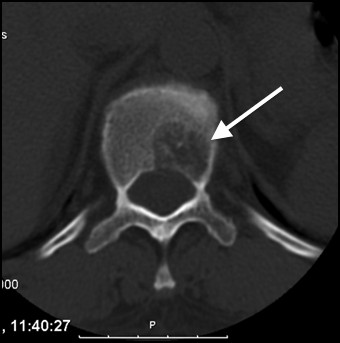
A well delimited lytic lesion on the left side of T11 body was observed on CT scan. This lesion conditioned a narrowing of the vertebral canal, with slight compression of the spinal cord. Arrow indicating the lesion.
